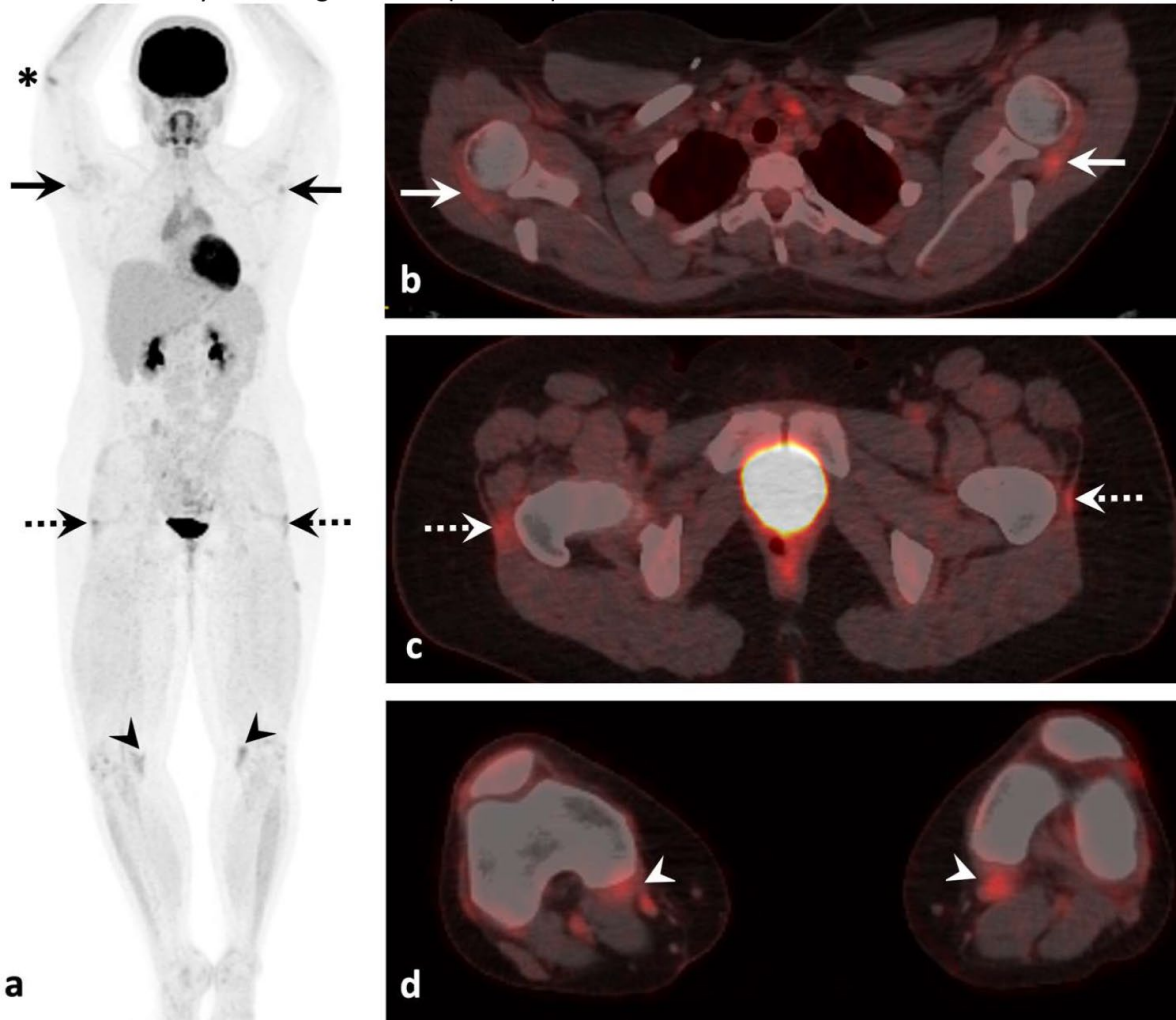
Whole body PET-CT following initiation of immunotherapy. Maximum intensity projection (a) and axial fused images (b-d) demonstrates symmetric increased metabolic activity at the bilateral supraspinatus (*solid arrows*), gluteus medius/minimus (*dashed arrows*), and semimembranosus (*arrowheads*) tendon insertions. There is also focally increased metabolic activity at the right elbow (*asterisk*)
